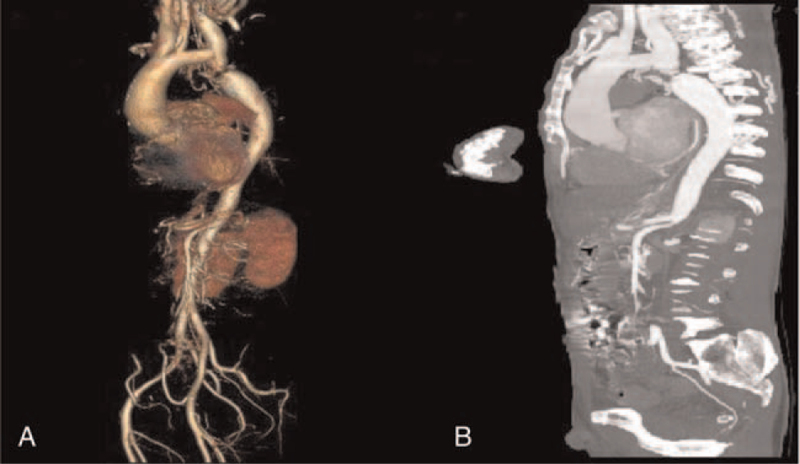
(A, B) Computed tomography angiography of aortic arch confirmed the diagnosis of interrupted aortic arch again.
